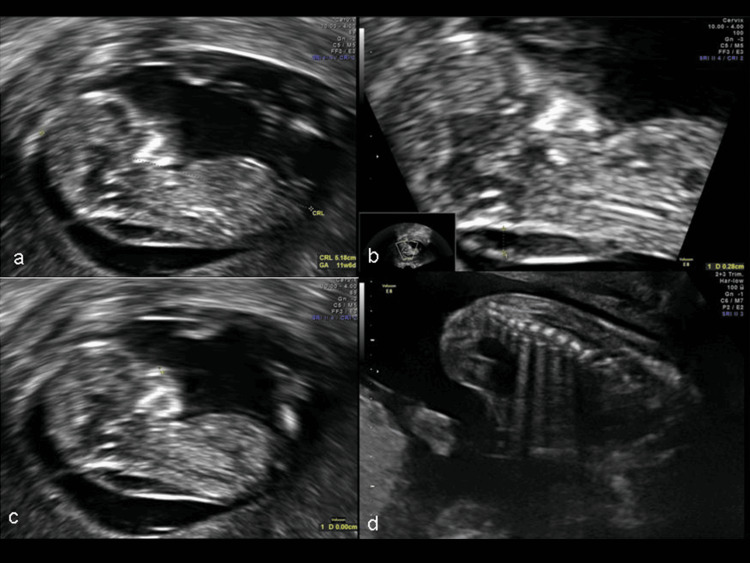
Ultrasound scan of the foetus at 11+6 weeks (a-c) and at 22+6 weeks (d). (a)Crown Rump Length (CRL), (b) nuchal translucency (NT), (c) nasal bone, (d) anatomy of ribs
